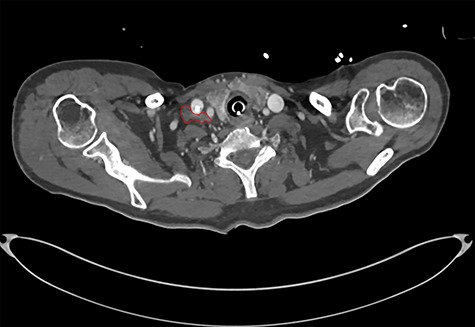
CT showing haematoma in the right carotid sheath following insertion of central line.
